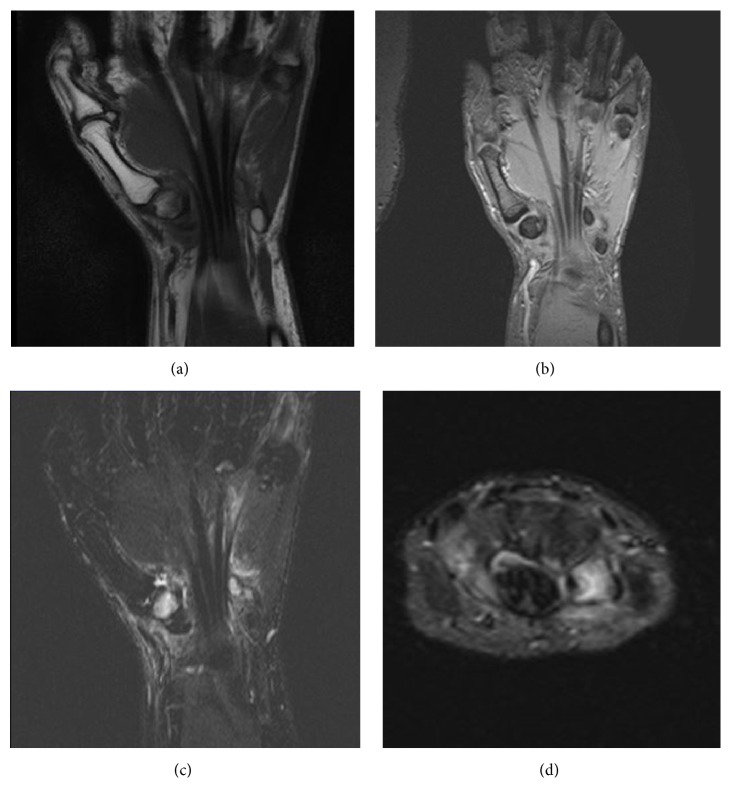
(a) T1-weighted coronal image reveals areas of low signal intensity affecting the trapezium. (b) PD coronal image shows heterogeneous signal intensity with areas of high signal intensity. ((c) and (d)) STIR coronal and axial images reveal increased signal intensity affecting the whole trapezium consistent with hyperemic areas due to repair process and oedema.
